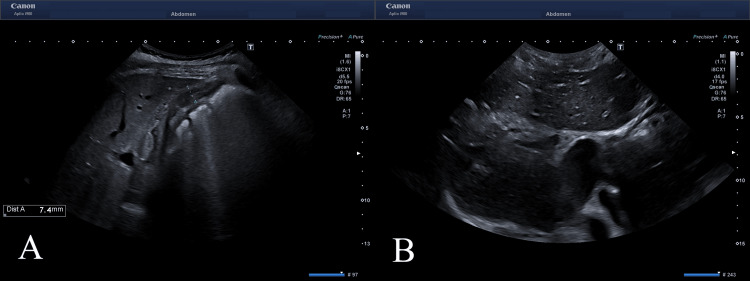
Ultrasound of abdomen. (A) Diffuse gall bladder wall edema along with hypoechoic hepatic parenchyma giving starry sky appearance, suggestive of presence of Hepatitis. (B) An enlarged liver with starry sky appearance.
